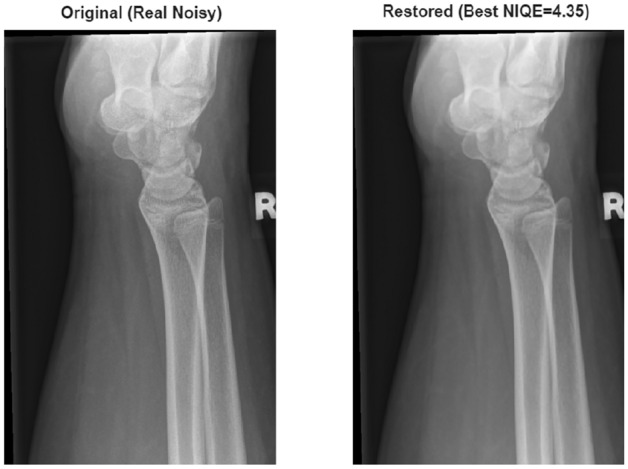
GRAZPEDWRI-DX X-ray dataset sample result.
